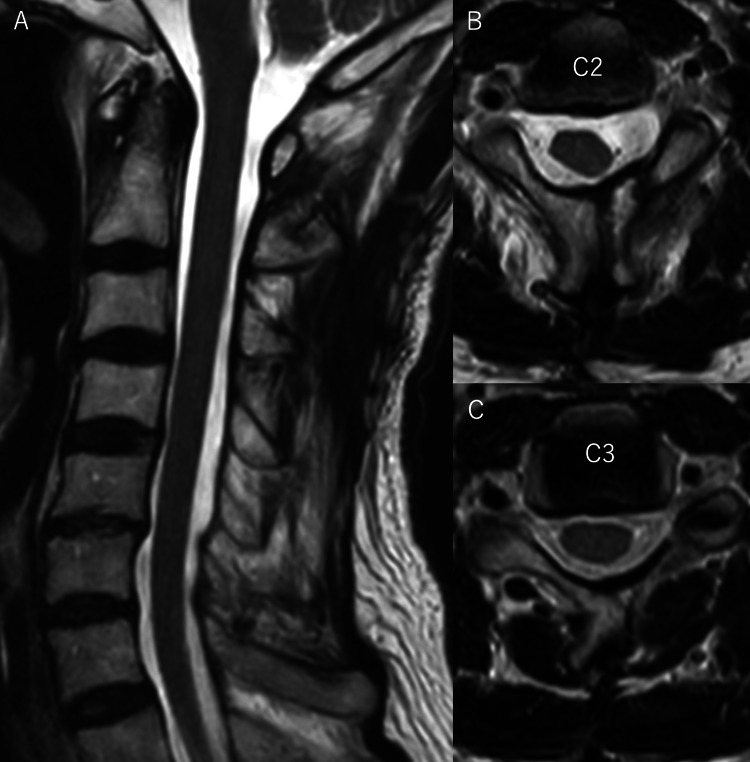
Postoperative MRI of case 2 (A) T2-weighted sagittal section showed that the spinal cord tumor had been completely removed, and the spinal structure had been well preserved. (B) T2-weighted cross-sectional image at C2 level showed that the spinal cord tumor had been completely removed and no muscle damage of C2 had occurred at all. (C) T2-weighted cross-sectional image at C3 level showed that the spinal cord tumor had been completely removed, and no muscle damage of C3 had occurred at all. In this case, we could completely remove the spinal cord tumor without causing any muscle damage.
